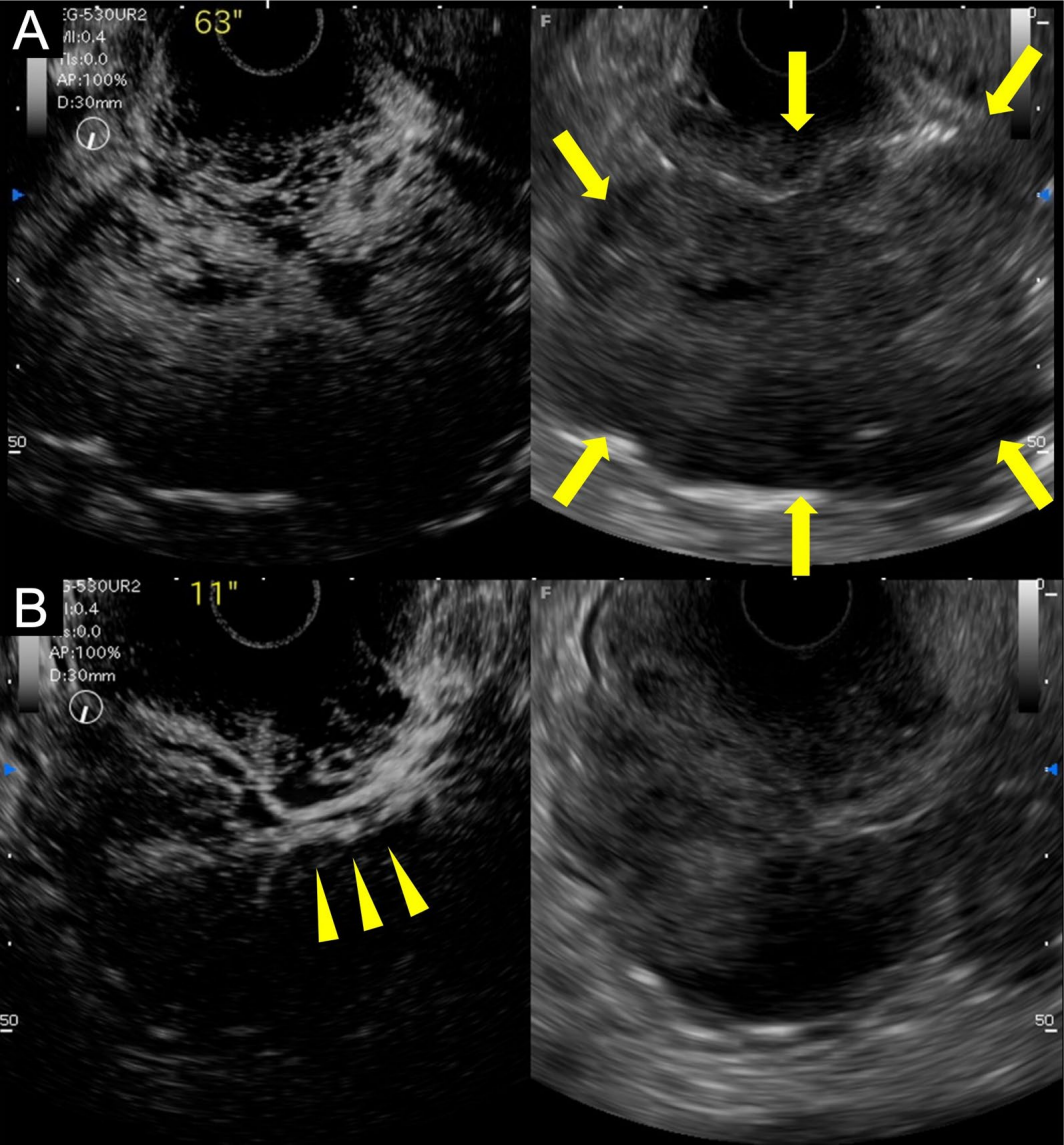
The left side is the contrast-applied image of the right-side image. A Endoscopic ultrasound showed heterogeneous internal echoes with partially mottled areas (arrows). B Applied contrast revealed rapid blood flow from the vessels at the stem (arrowheads), clarifying its internal structure
